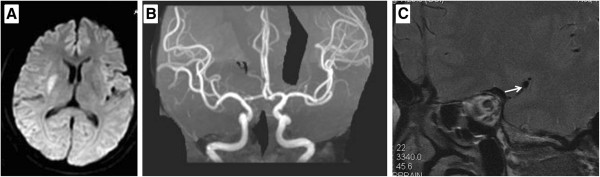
MRI, MRA and HR MRI findings of Case 2. (A) MRI diffusion weighted image shows a hypersensitive lesion on posterior limb of internal capsular. (B) MR angiography of the MCA is normal. (C) High-resolution MRI of MCA indicates the presence of an atherosclerotic plaque on the frontal wall of the right proximal MCA.
